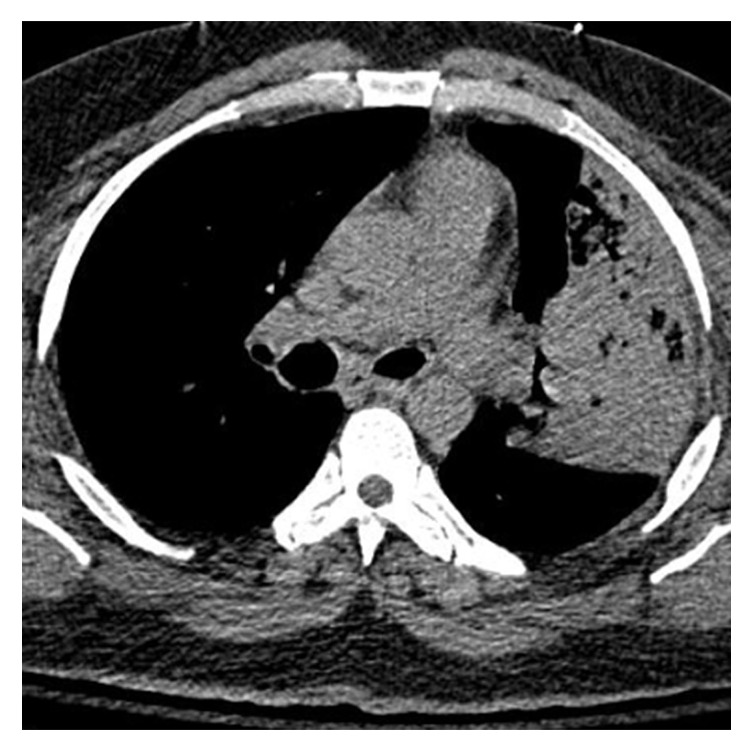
Axial section chest CT showing consolidation in the left lingual with air bronchograms consistent with lobar pneumonia, without pleural effusion.
